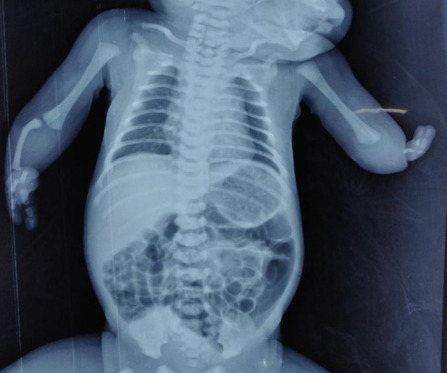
X-ray image of the child with congenital upper limb deficiency.
